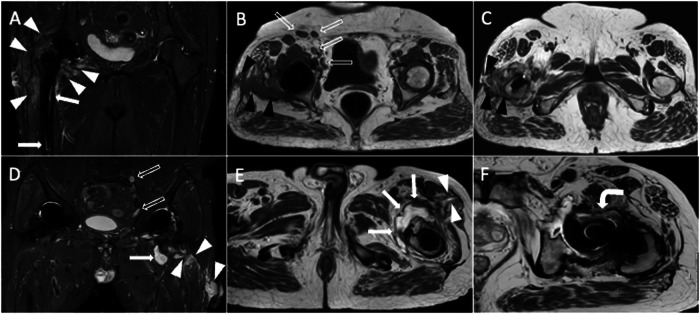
Two cases of infection of the right THA of a 65-year-old female (A–C) and the left THA of a 73-year-old male (D–F). Coronal STIR (A) image shows pericapsular edema (white arrowheads) and femoral periprosthetic bone edema (arrows). Axial T1-weighted (B) and axial T2-weighted (C) images show a sinus tract that communicates with the skin (black arrowheads). Increased number and size of loco-regional inguinal lymph nodes (void arrows, B) can be observed too compared to the contralateral side. Coronal STIR (D) and axial T2-weighted (E) images show a periprosthetic fluid collection (arrows) with the sinus tract up to the skin (arrowheads). Also note lamellated synovitis (curved arrow) anterior to the prosthetic neck on axial T2-weighted image (F) and enlarged loco-regional iliac lymph nodes on coronal STIR image (void arrows, D)
